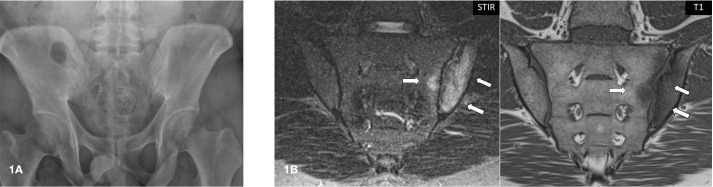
(A) Radiograph of the SIJ from a patient with nr-axSpA without pathological findings. (B) MRI of the same patient. Oblique coronal STIR and T1-weighted images. Pronounced bone marrow oedema on the left SIJ (white arrows), shown as hyperintense signal on STIR and hypointense signal on T1-weighted MR, especially at the iliac bone.
